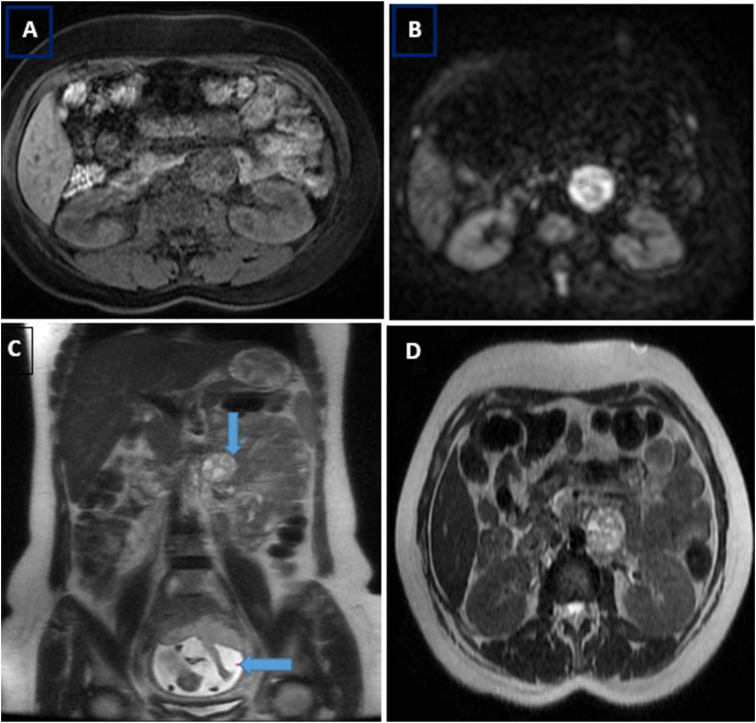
Abdominal MRI in sequences: Axial T1 (A), axial diffusion b1000 (B), coronal (C) and axial T2 (D) showing a round mass, well-limited, retroperitoneal left lateroaortic in isosignal T1, hypersignal T2 and diffusion, containing areas of fluid signal measuring 36 mm in diameter. Note: a gravid uterus.
